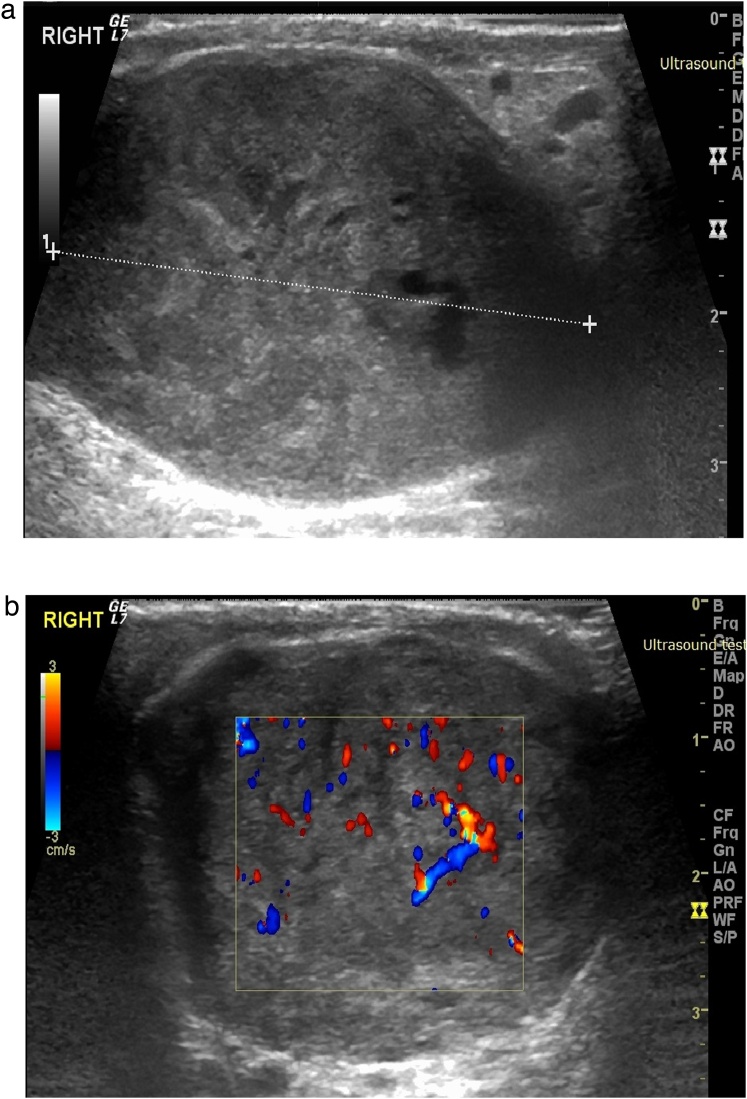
A: Scrotal ultrasound showing a well-circumscribed mass of mixed heterogeneous echogenicity on the right side. B: Doppler ultrasound demonstrating increased vascularity of the lesion.
